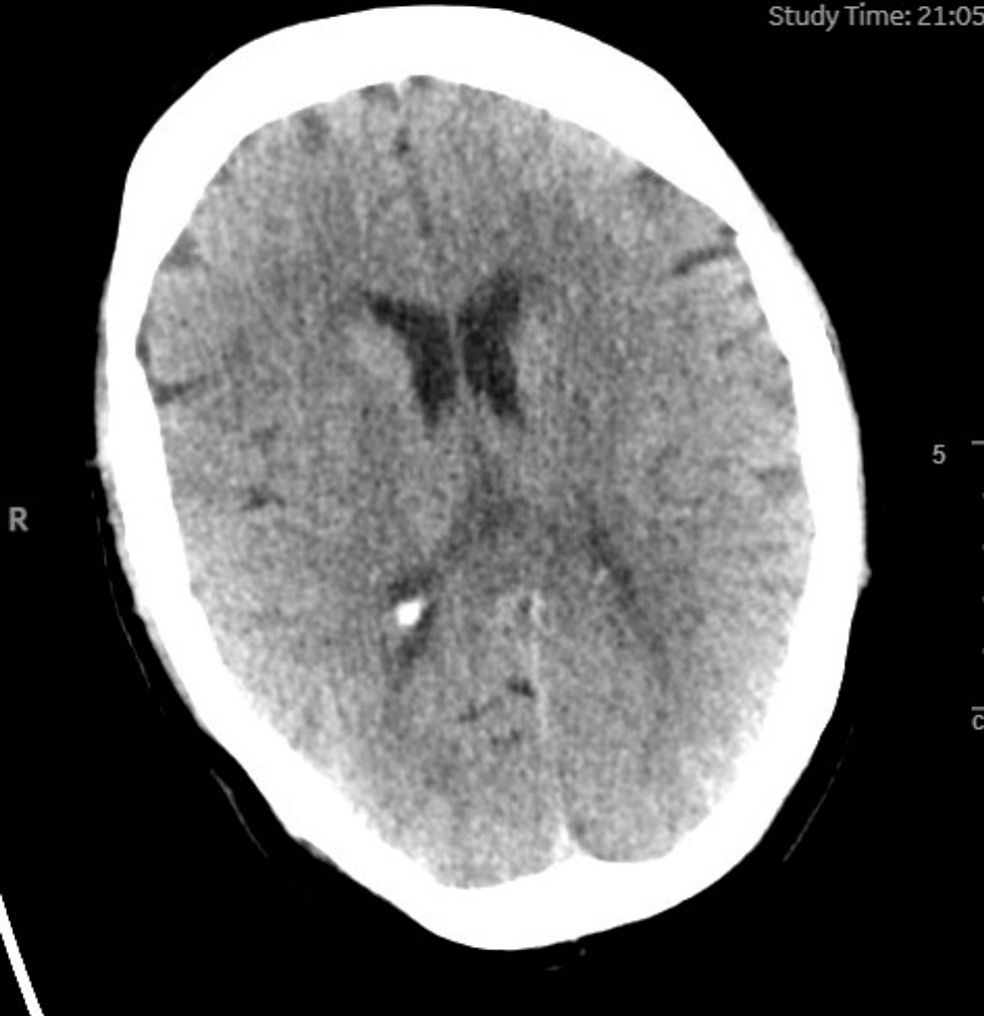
Non-contrast CT head demonstrating no abnormalities
